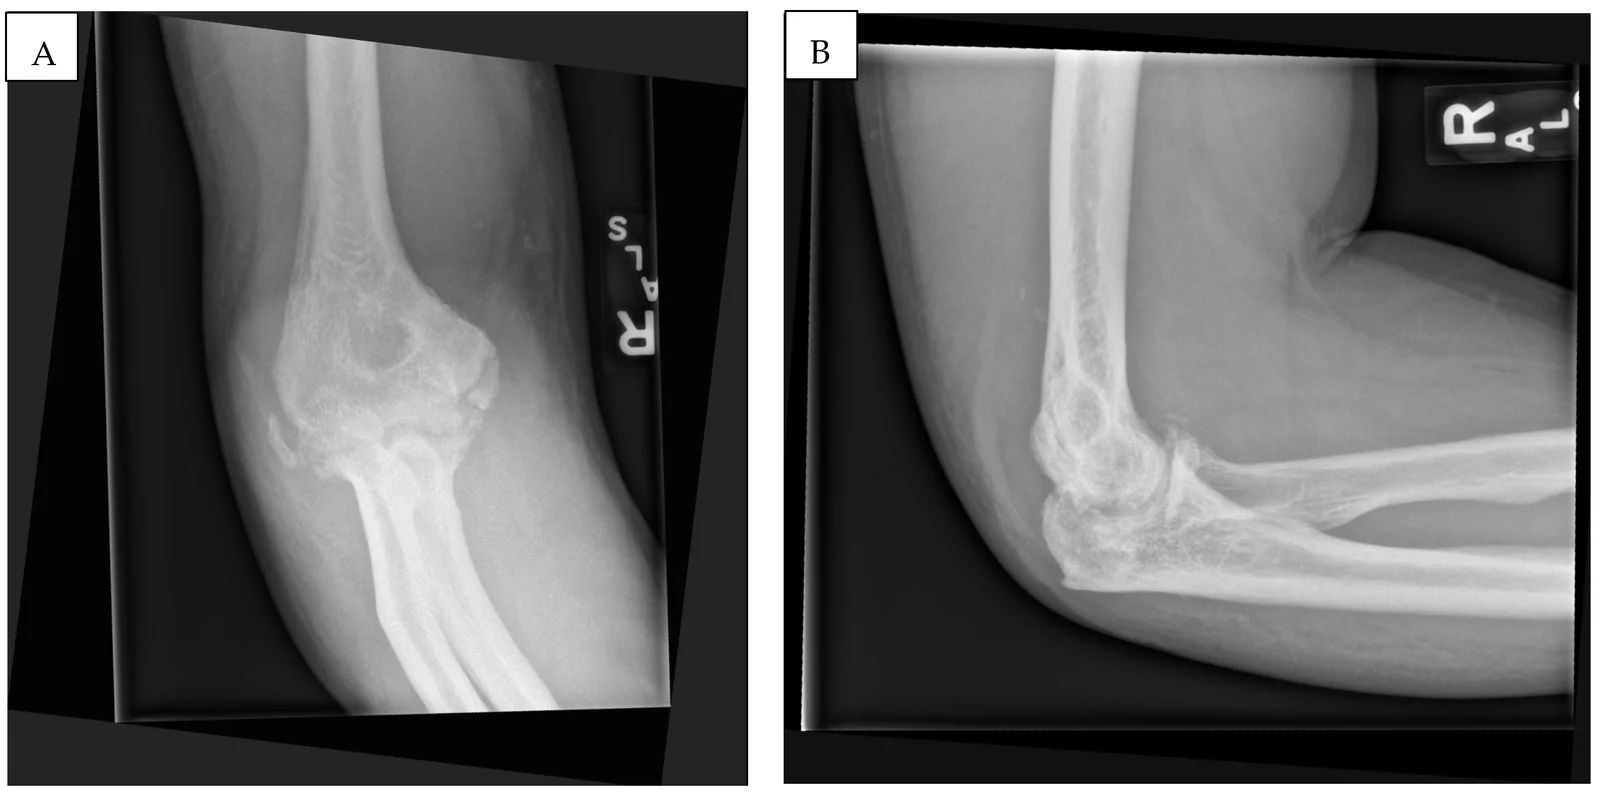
Postoperative (A) anterior to posterior (AP) radiograph. (B) Lateral radiograph. This figure demonstrates satisfactory elbow alignment and no new HO formation at final follow-up.
